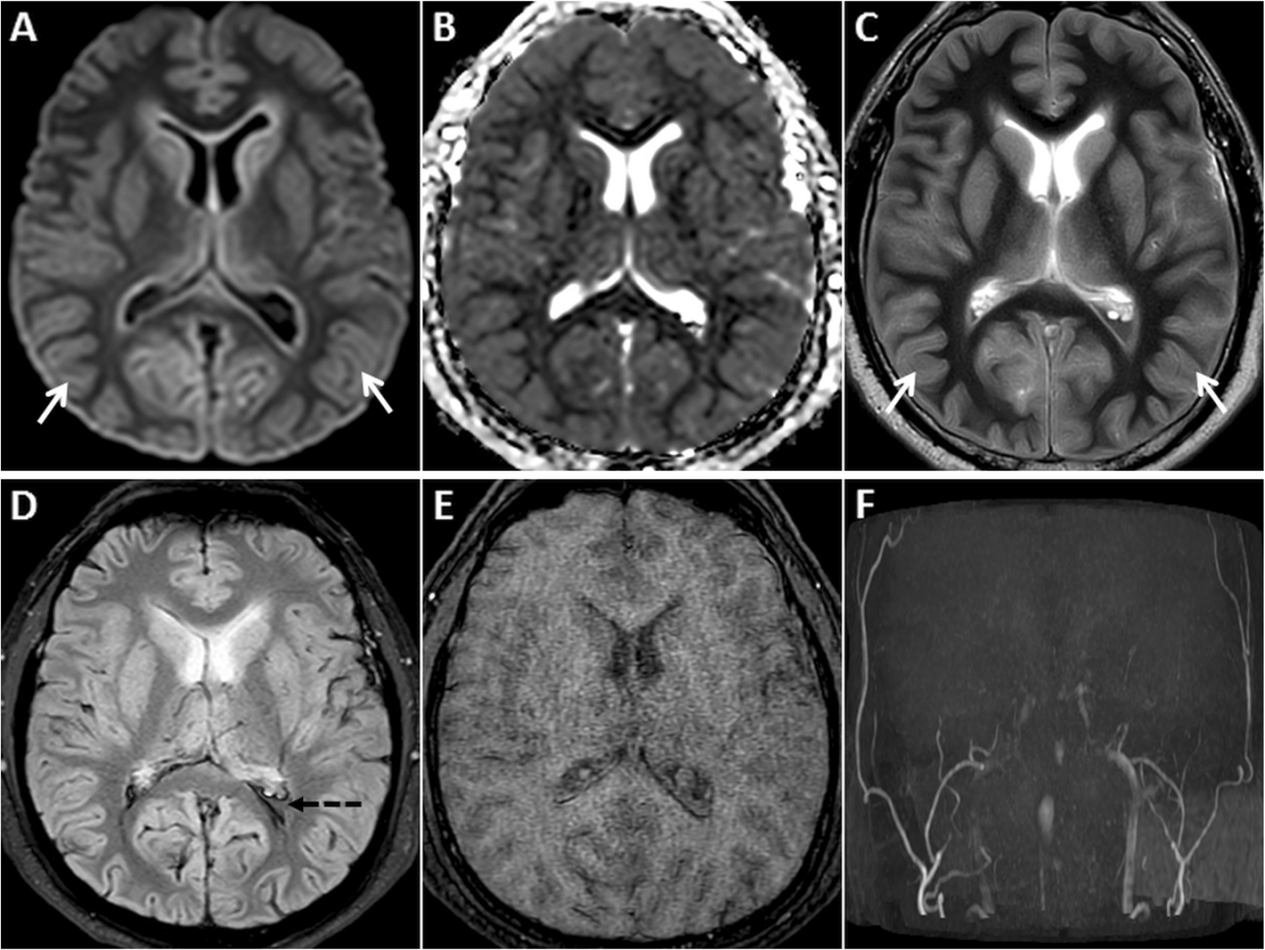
MRI of the brain performed in the subacute phase of HIE. DWI hyperintensity is noted in the deep grey nuclei and extensively in the cerebral cortices (arrows) on axial DWI image (a). There is no signal drop on axial ADC image (b). Diffuse cortical swelling and signal changes (arrows) are appreciated better on the axial T2W image (c). Minimal intraventricular blood (dashed arrow) is seen on axial GRE image (d). Axial source 3D-TOF MRA image (e) and reconstructed maximum intensity projection MRA image of Circle of Willis (f) show no flow signal in the intracranial arteries
